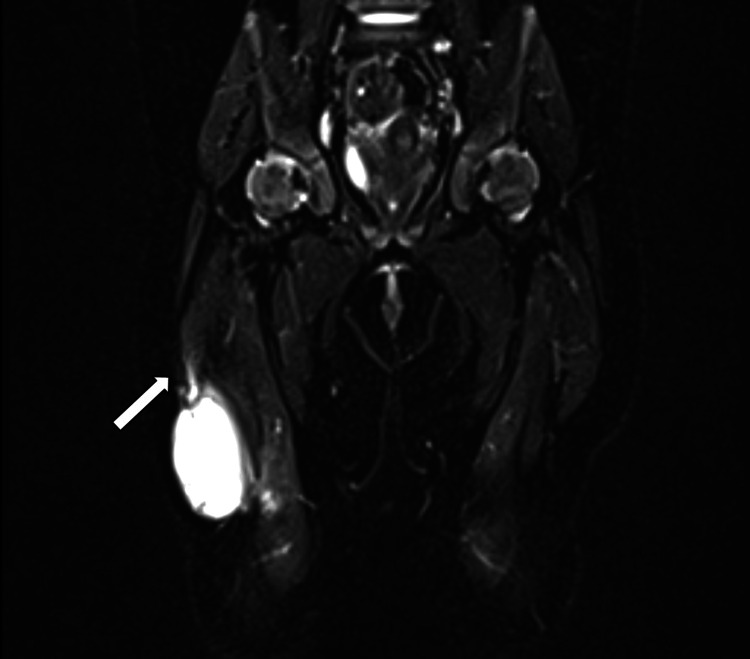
T2-weighted MRI of the thigh, coronal view. A cystic mass is visualized along the anterolateral aspect of the distal thigh, measuring 40 mm × 14 mm × 22 mm in craniocaudal, transverse, and anteroposterior dimensions. The mass has a broad base against the vastus lateralis with infiltration into the muscle (arrow).
